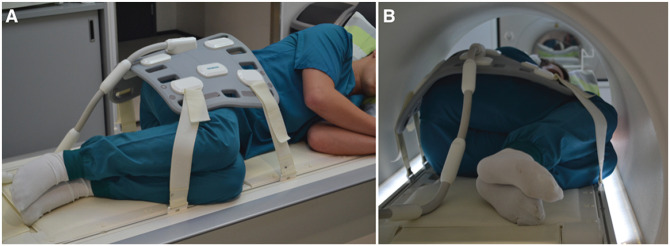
The novel magnetic resonance imaging scanning position of hip flexion (A) before and (B) during the scan in a patient who is 176 cm tall (body mass index, 23.9).
